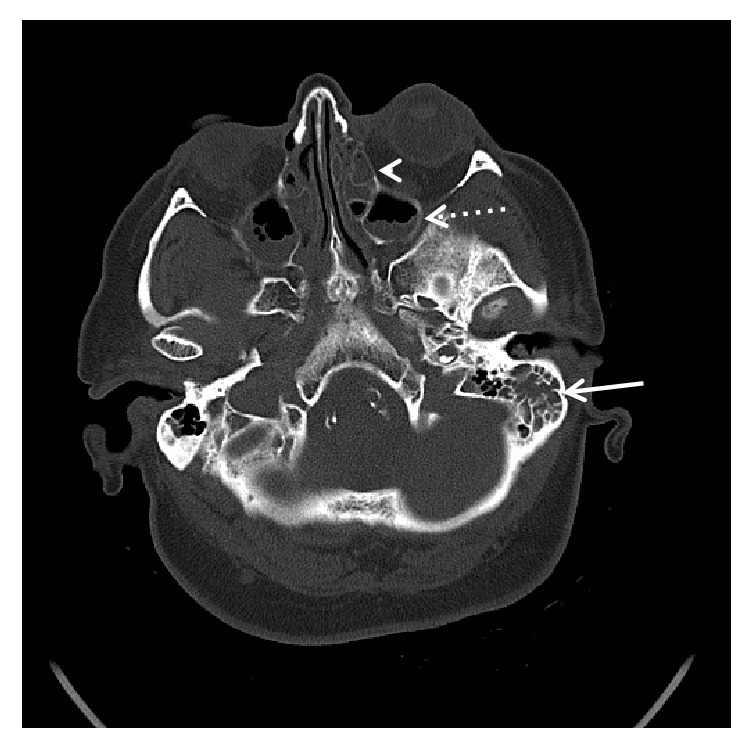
Axial CT demonstrating opacification of the mastoid air cells (solid arrow), ethmoid sinuses (arrowhead), and maxillary sinuses (dashed arrow) in keeping with mastoiditis and sinusitis.
